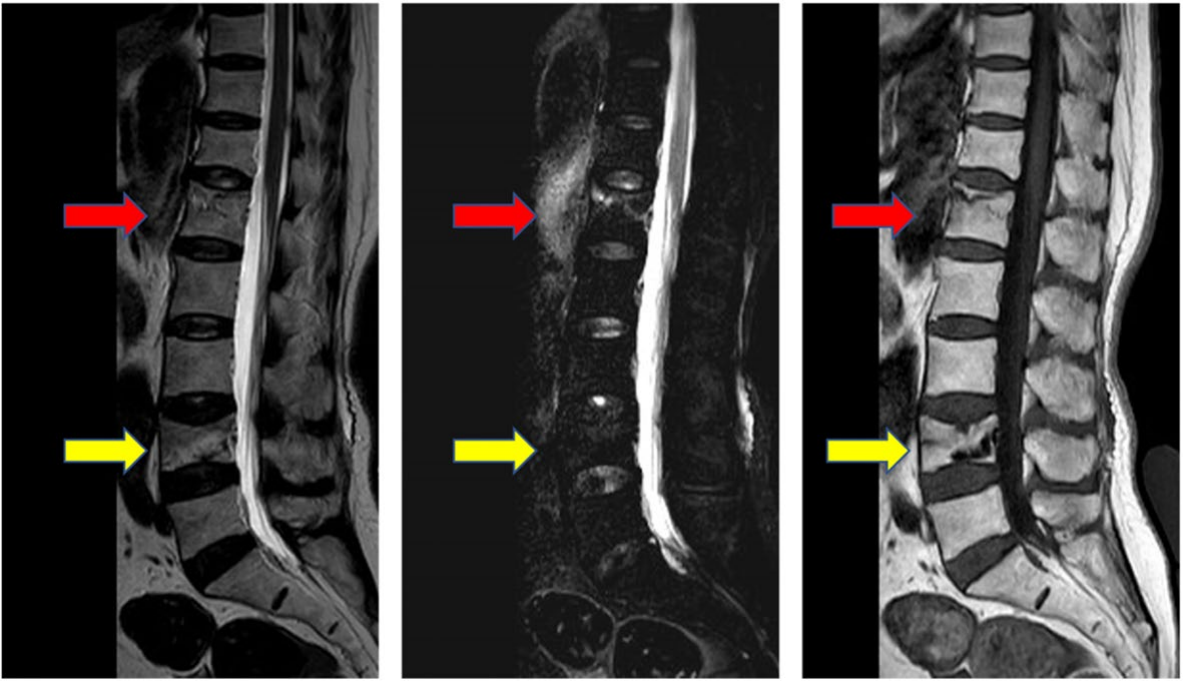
MRI example of vertebral refracture. Magnetic resonance imaging showed an old L4 fracture and a recurrent fracture of the L1 vertebral body in an 84-year-old female
